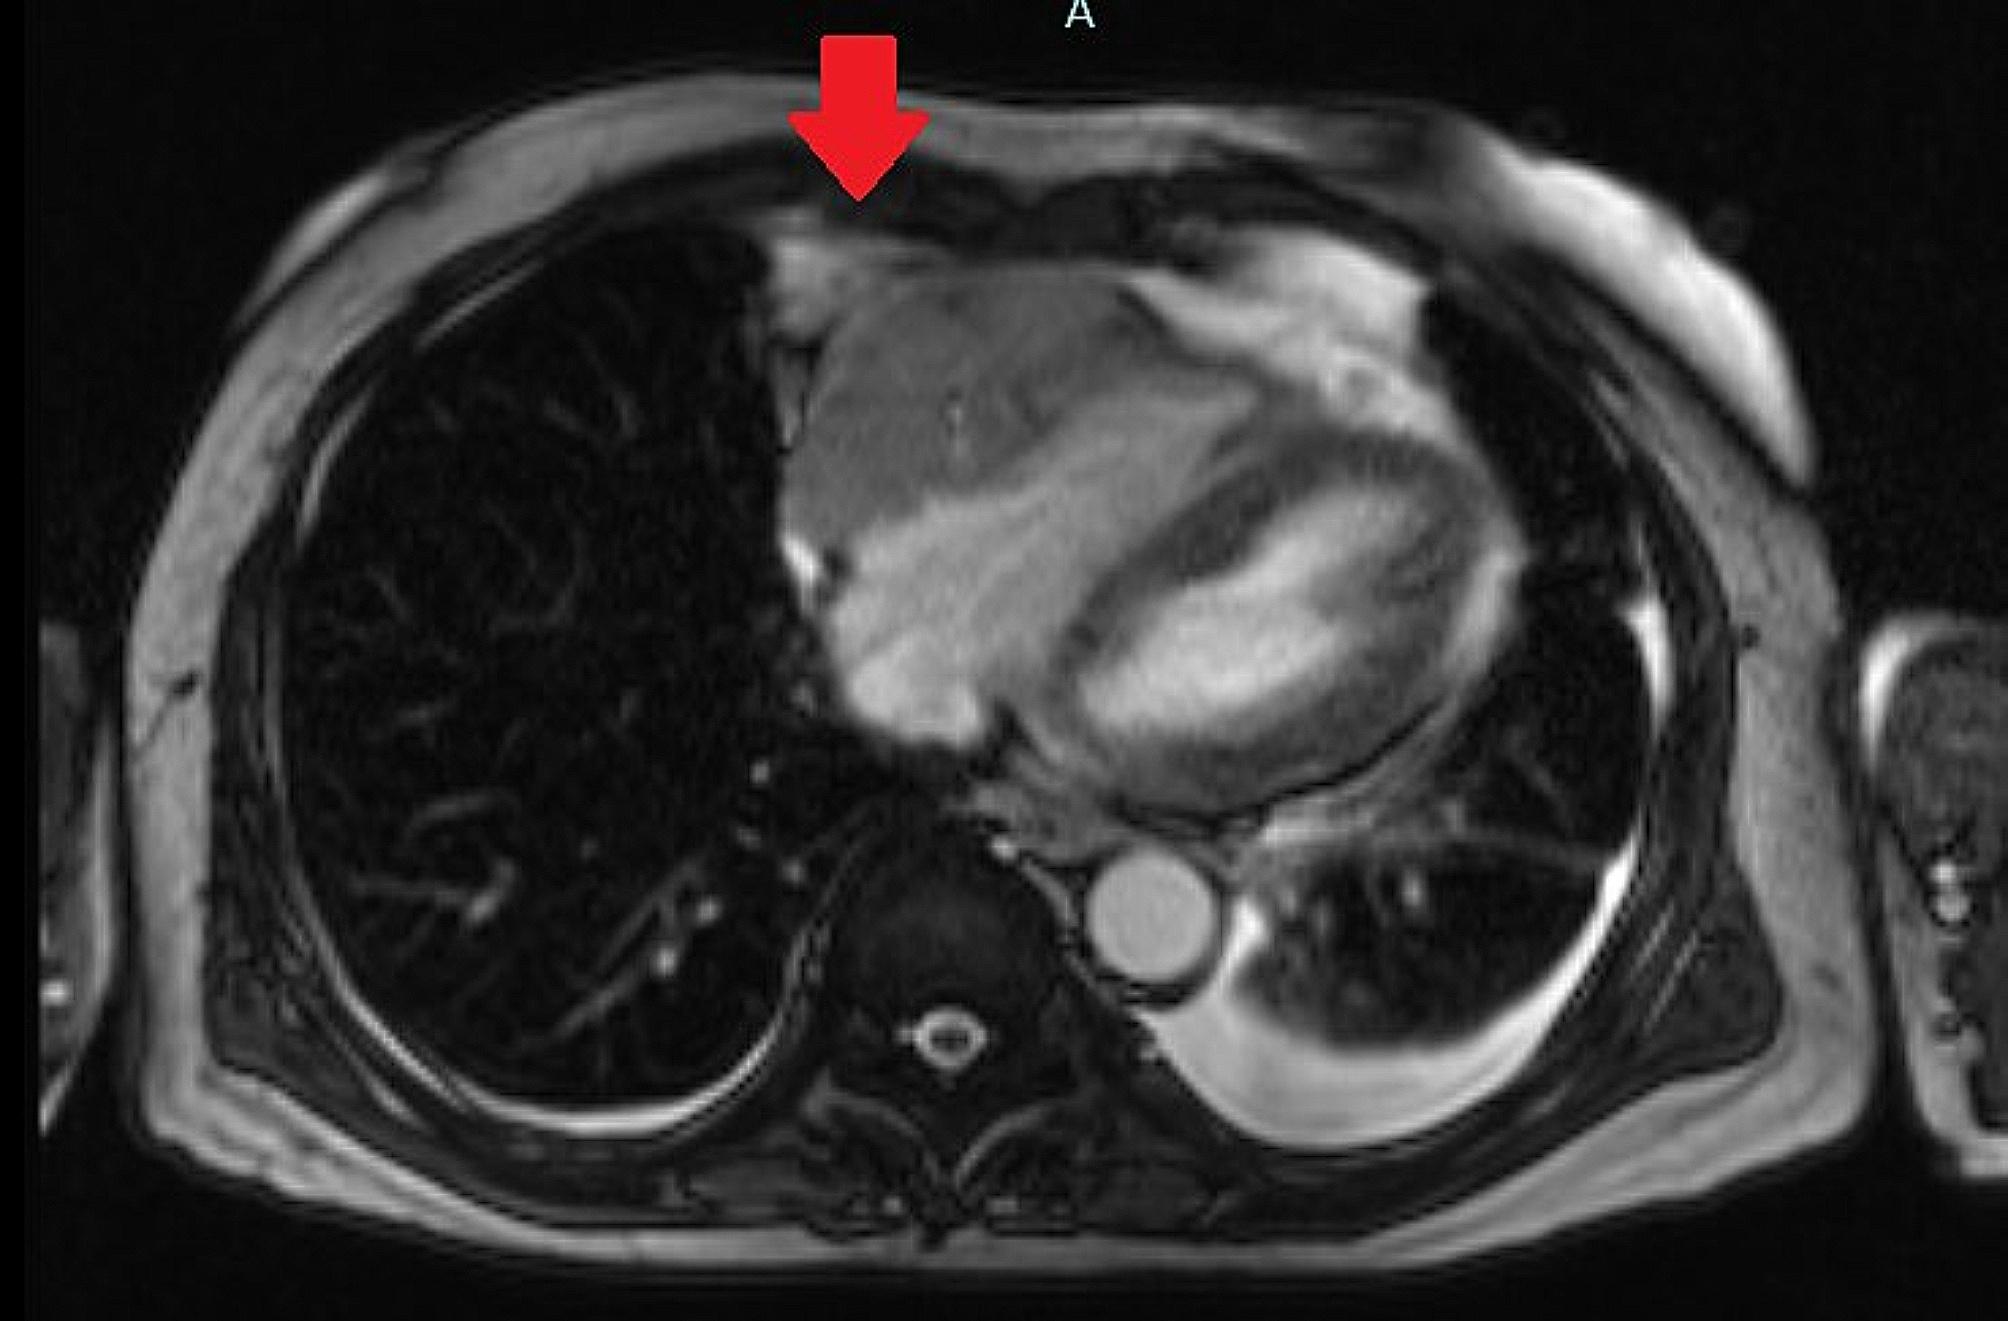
Cardiac magnetic resonance imaging showing mass from right atrioventricular groove
